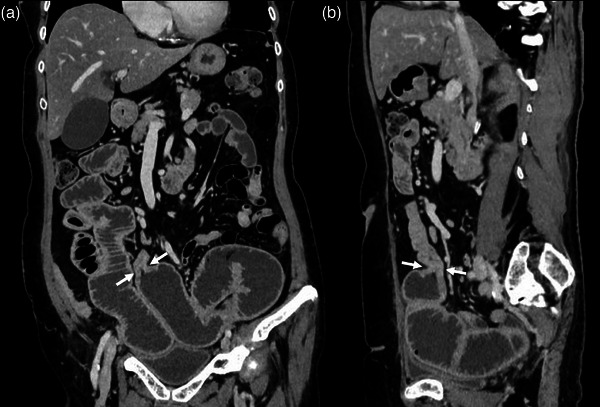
Contrast‐enhanced abdominal computed tomography showing a small bowel wall thickening with proximal loop dilatation (a: coronal image, b: sagittal image, arrows).
